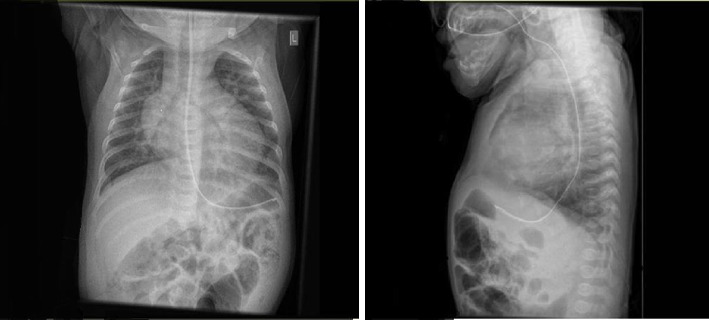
Chest x-ray showed prominence of the cardiothymic silhouette pulmonary vascularity and increased interstitial marking.
